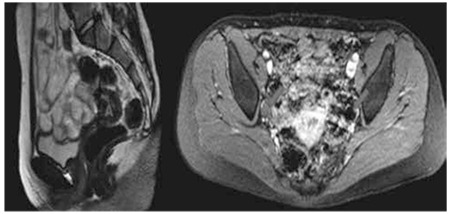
Magnetic resonance imaging of uterus (A) and gonads (red arrows).
